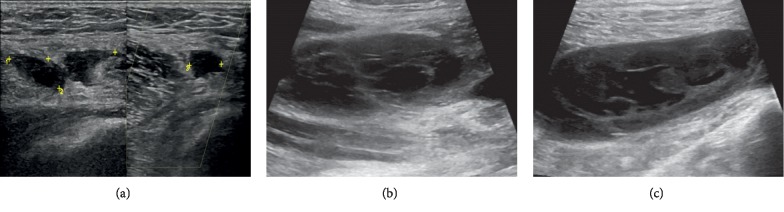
Ultrasound (US) of the left lower extremity: (a) Baker's cyst. (b) Follow-up US showing the increased size of baker's cyst. (c) Image depicting ruptured Baker's cyst.
